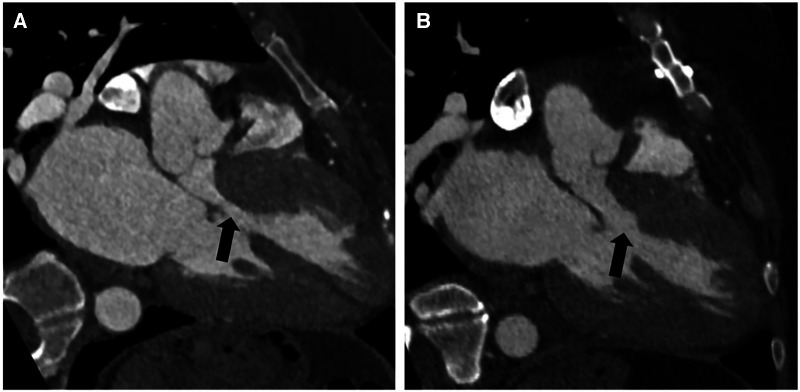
Preoperative contrast-enhanced CT angiography with a three-chamber view during diastole showing asymmetric thickening of the basal septum with narrowing of the left ventricular outflow tract (black arrow) (A). Postoperative contrast-enhanced CT angiography with a three-chamber view shows changes from septal myectomy with significantly reduced narrowing of the left ventricular outflow tract compared to preoperative (black arrow) (B).
